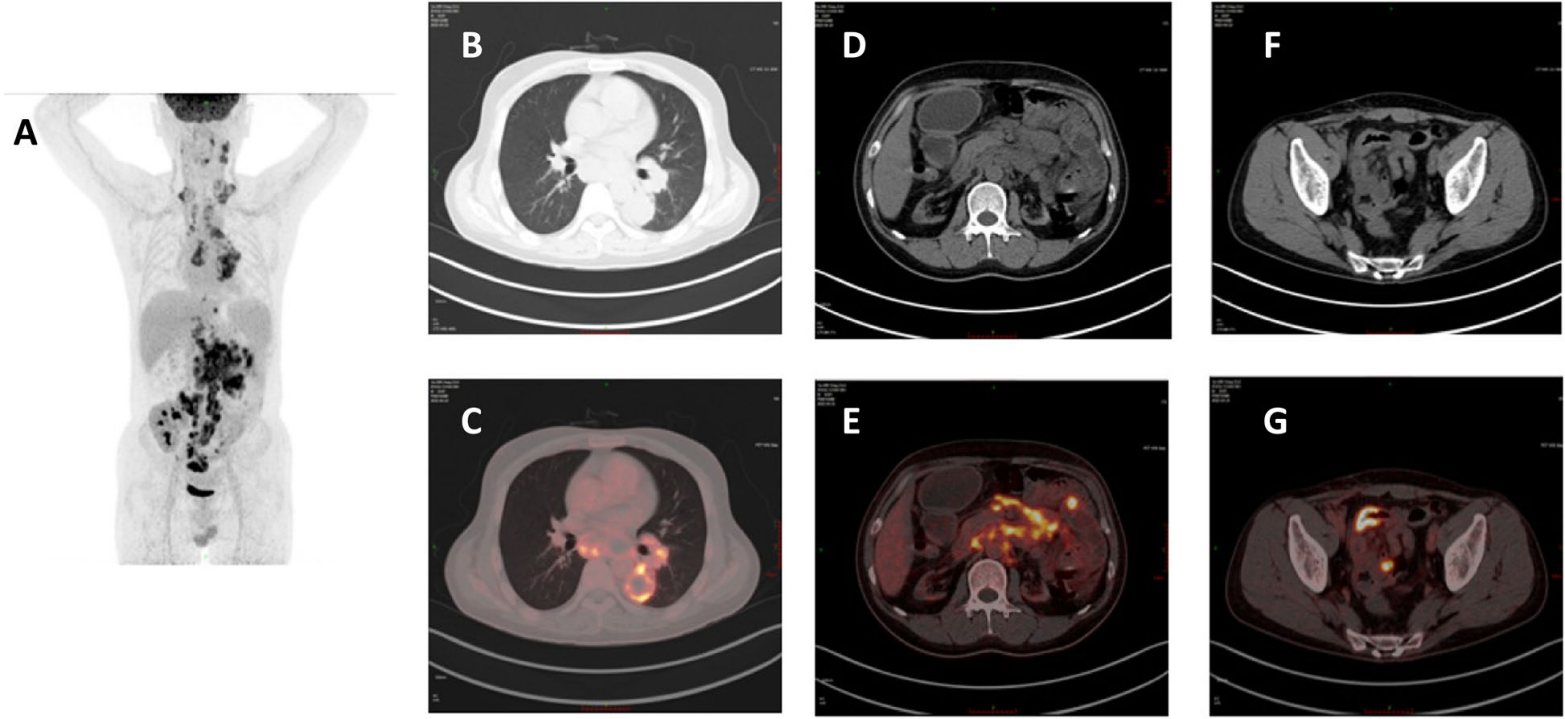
Head-Body PET Examination + Glucose Measurement (18F-FDG) (A, C, E, G) showed multiple lymph nodes were enlarged, the left hilar and mediastinal lymph nodes were enlarged, soft tissue density clumps of the dorsal and posterior basal segments of the left lower lobe, (B, C), multiple lymph nodes were enlarged in the retroperitoneal and superior mesangial and mesangial roots (D, E), multiple parts of the small intestine in the abdominal-pelvic cavity are thickened and FDG metabolism increased abnormally (F, G)
